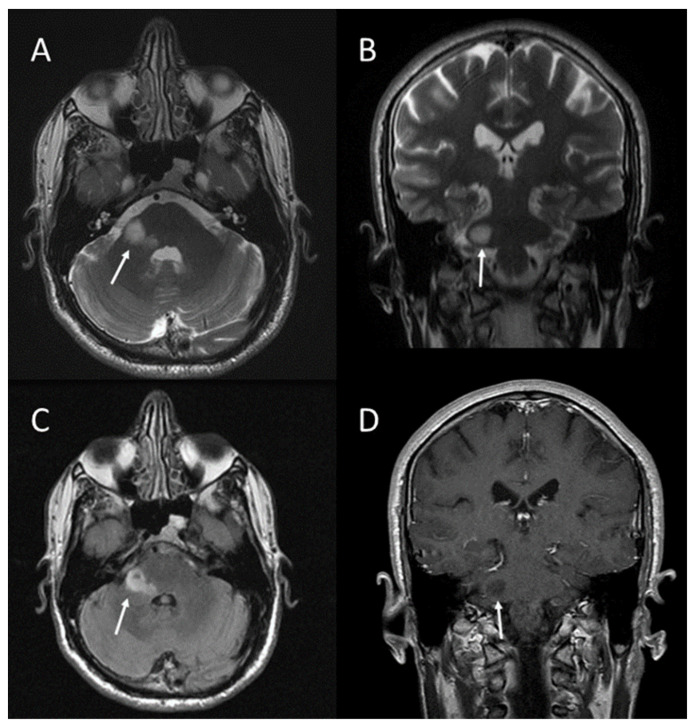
Focal area of signal intensity (FASI) in the right middle cerebellar peduncle (arrows). Axial T2-weighted FRFSE (A); coronal T2-weighted FRFSE (B); axial T2 FLAIR (C); coronal T1-weighted FSE after gadolinium administration (D) [11].
